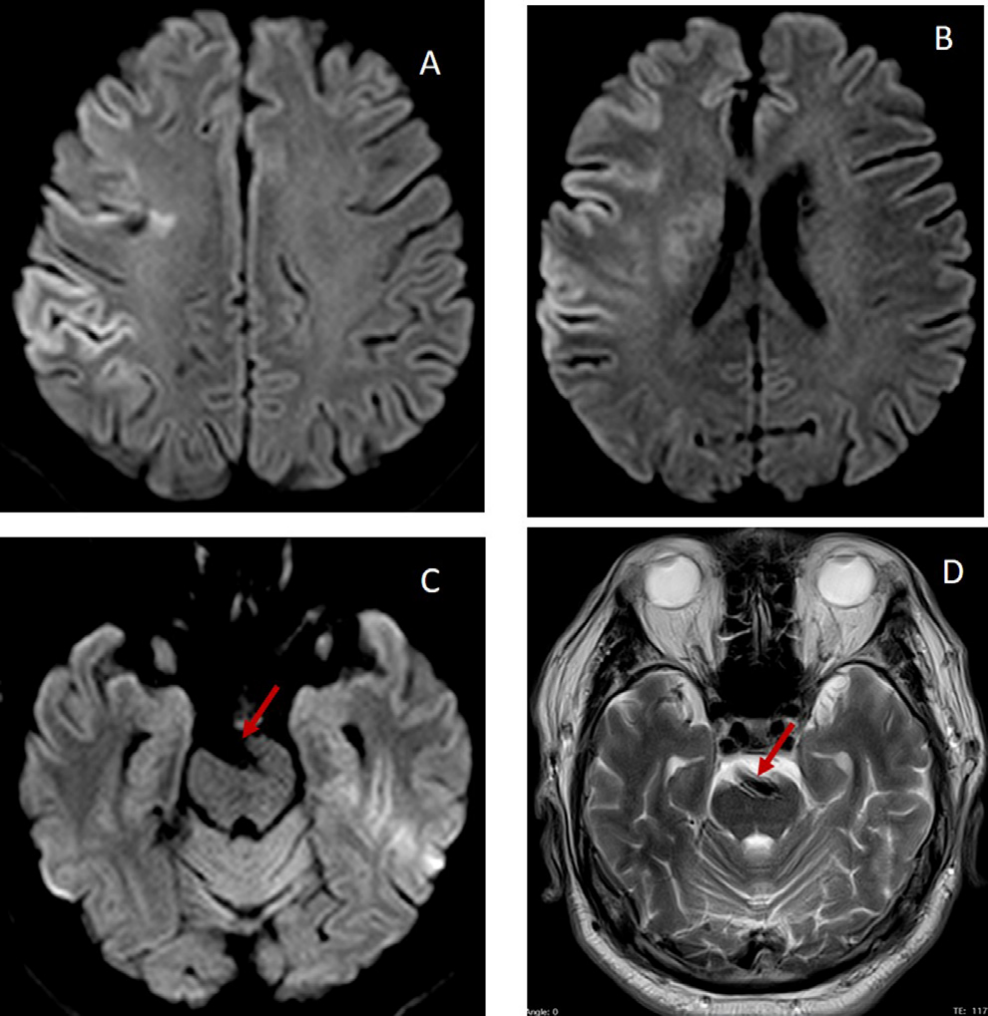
(A and B) Diffusion-weighted imaging (DWI) show an acute right frontal stroke. (C) DWI and (D) T2 show arterial compression on the pons (red arrow). (Color version of figure is available online.)
